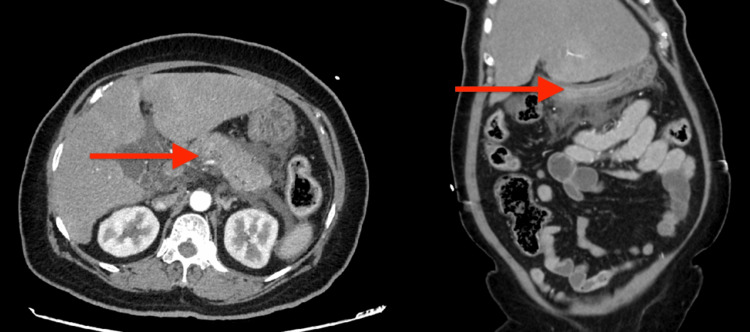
CTA showing acute interstitial edematous pancreatitis with pancreatic edema, peripancreatic fluid, and possible evolving necrosis at the neck. CTA: CT angiogram
